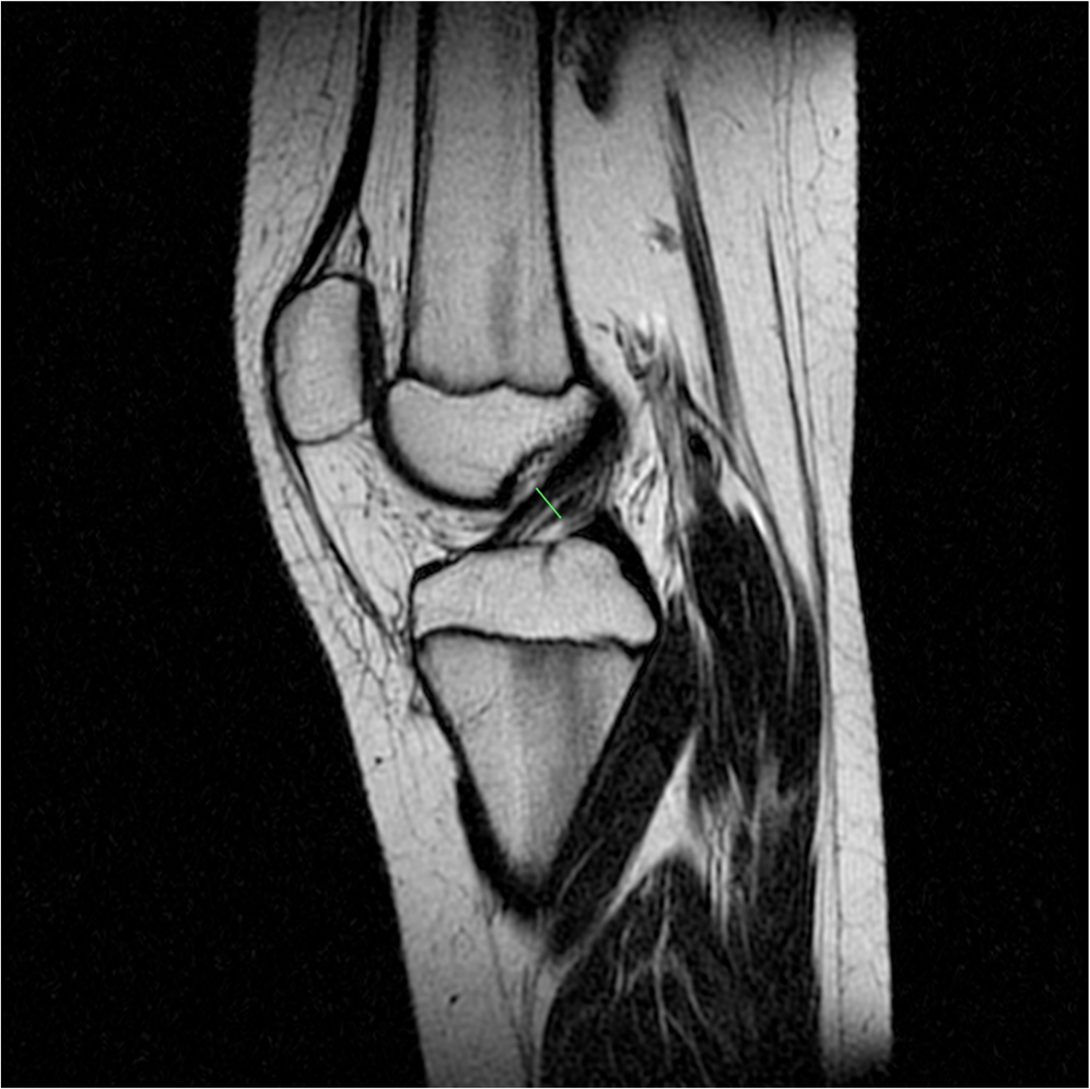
Knee MRI—measurement of the ACL diameter
